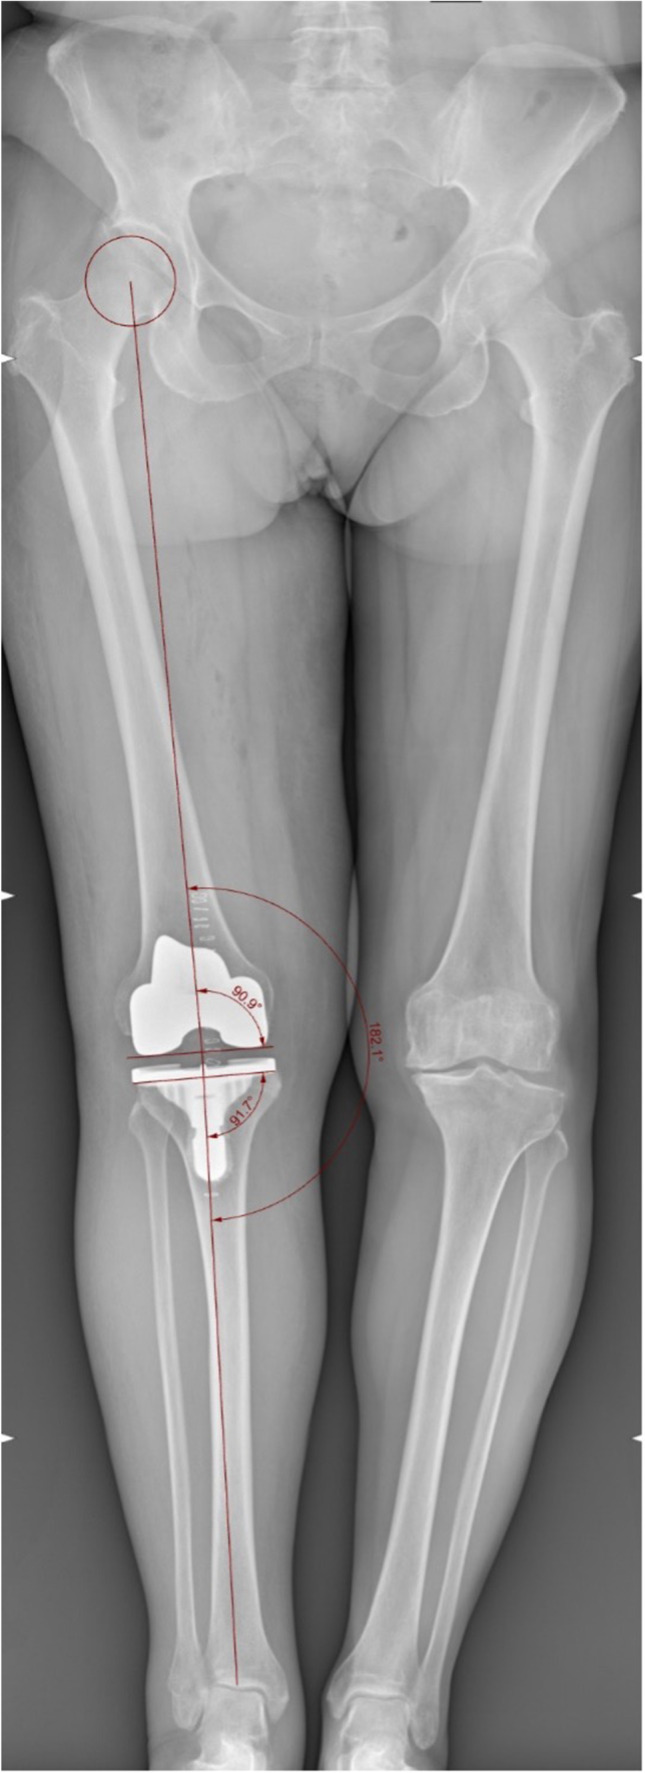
Post-operative x-ray measurement
